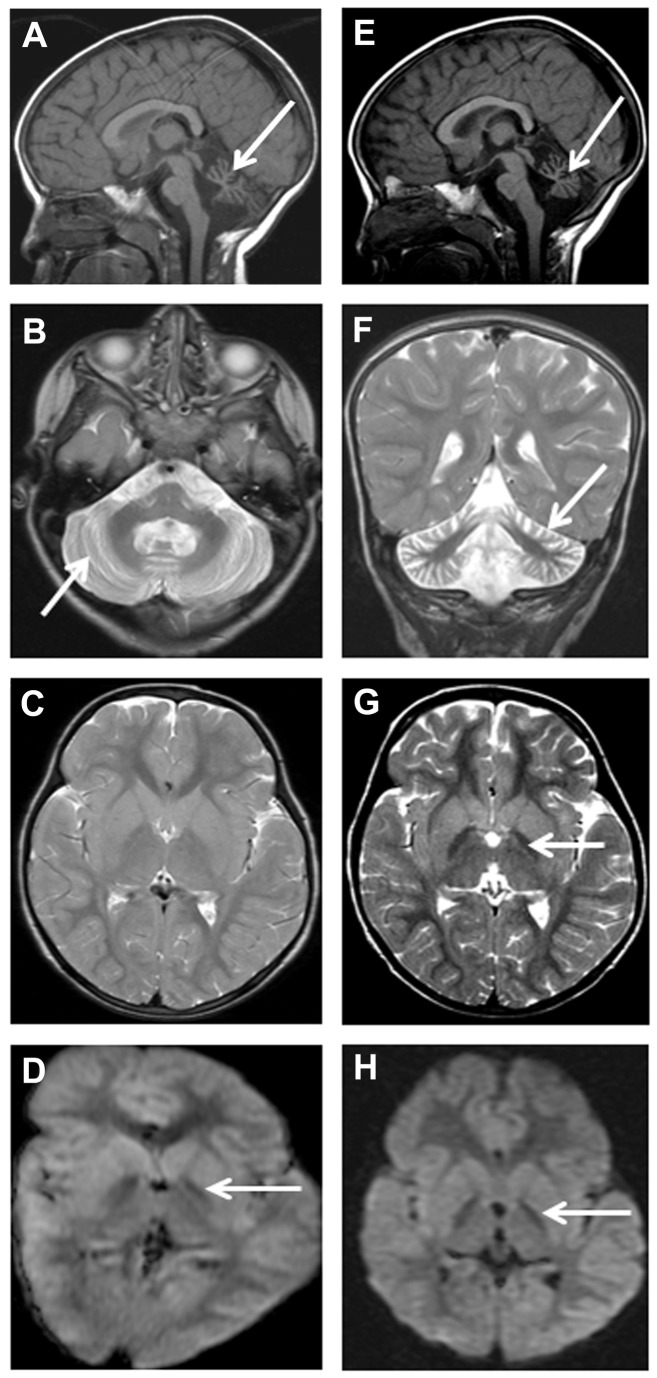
Patient F1 (P1) MRI, age 3 years 1 month (A-D), and 4 years 2 months (E-H). There is increased CSF space around the cerebellum (arrows in A and E) associated with cerebellar cortical atrophy and gliosis, with widening of folia and increased signal in the residual cerebellar cortex (arrows in B and F). Axial T2-weighted (C and G) and axial diffusion (D and H) highlight iron deposition as reduction in signal intensity in the *globus*
